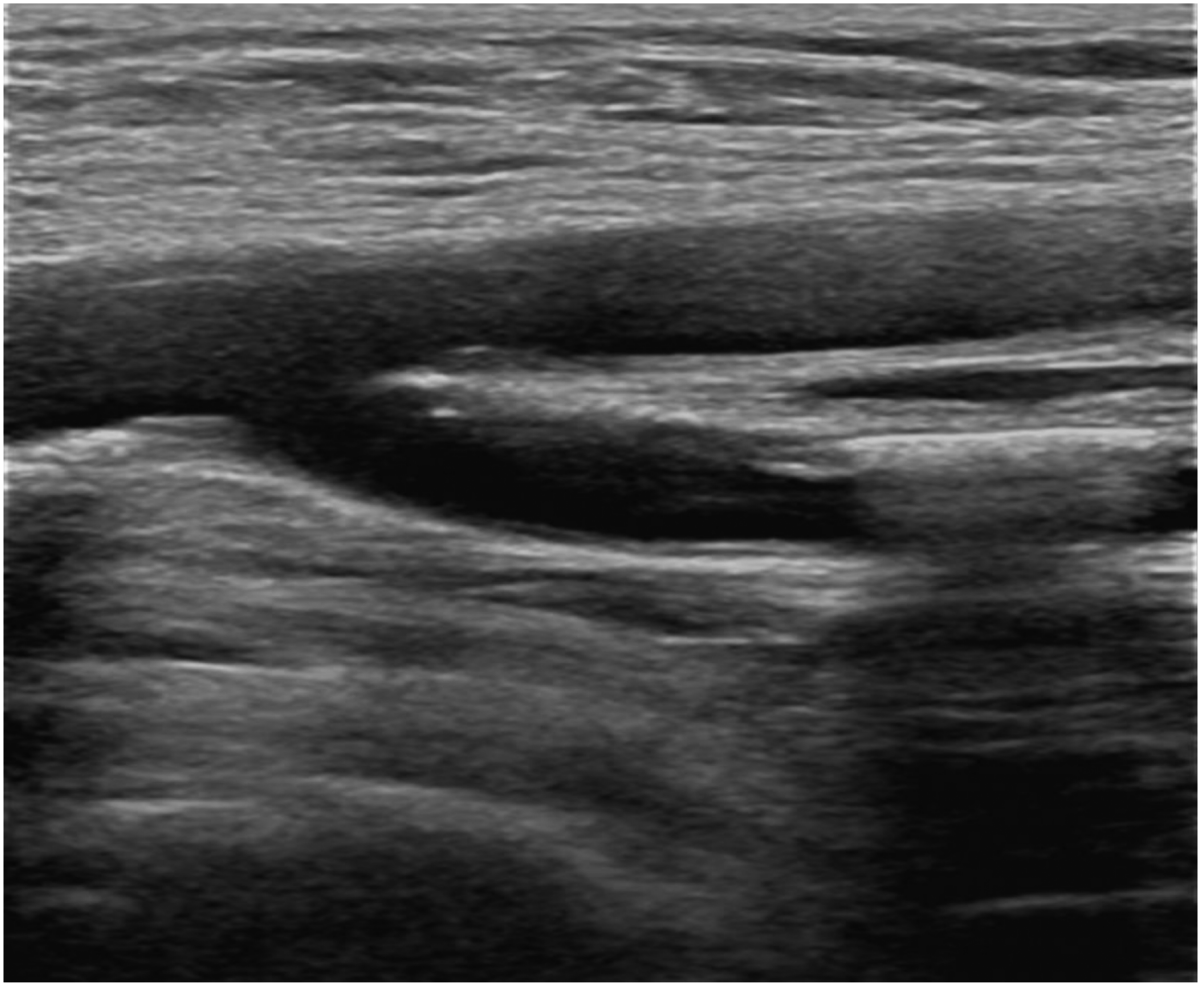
Lower extremity vascular ultrasound suggests lower extremity arterial thrombosis leading to arterial lumen occlusion.
